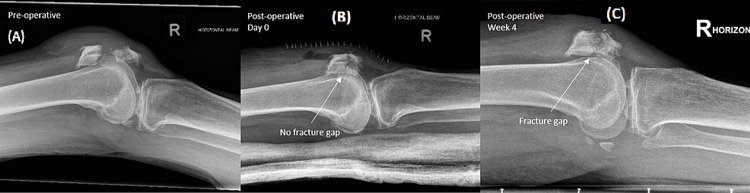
Radiograph of a mid-pole transverse patella fracture fixed with suture technique (A) Preoperative x-ray showing the mid-pole displaced transverse patella fracture. (B) Immediate postoperative x-ray after suture fixation of the patella fracture. (C) Four weeks postoperative x-ray showing fracture gap displacement of around 2 mm.
